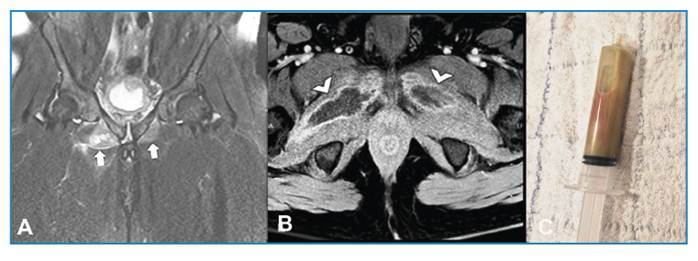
(A) Coronal fat-suppressed T2-weighted magnetic resonance imaging (MRI) of the pelvis demonstrating diffuse muscle edema in the bilateral external obturator muscles (arrows). (B) Axial fat-suppressed post-contrast T1-weighted MRI showing fluid collections in the muscle bellies, with bilateral peripheral enhancement (arrowheads). (C) Aspirated purulent material obtained during CT-guided percutaneous drainage, confirming the diagnosis of pyomyositis caused by oxacillin-sensitive *Staphylococcus*.
